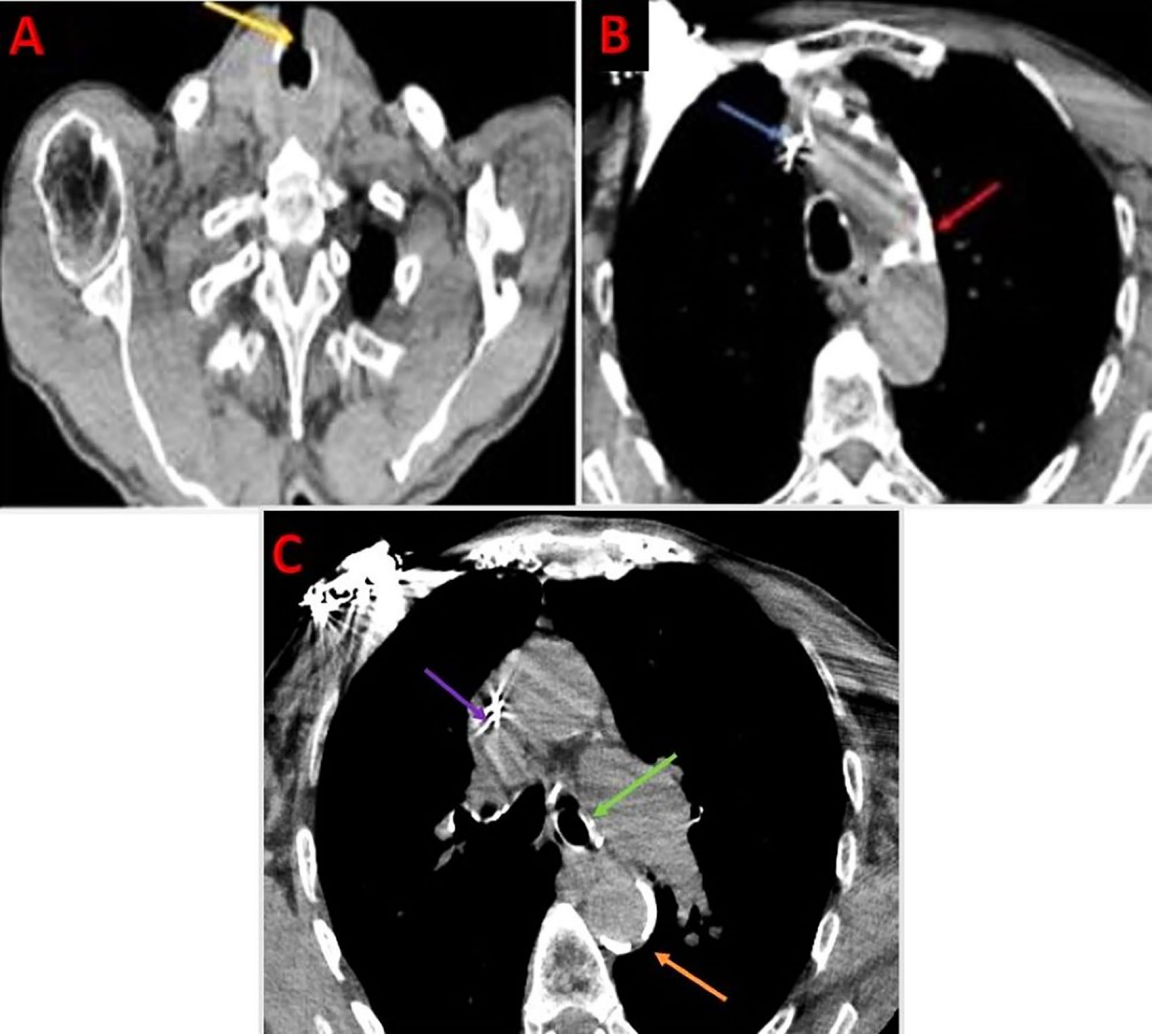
CT-Scan showing tracheostomy orifice (yellow arrow), misplaced Tube (green arrow), and vascular Calcifications (red/orange arrows).
